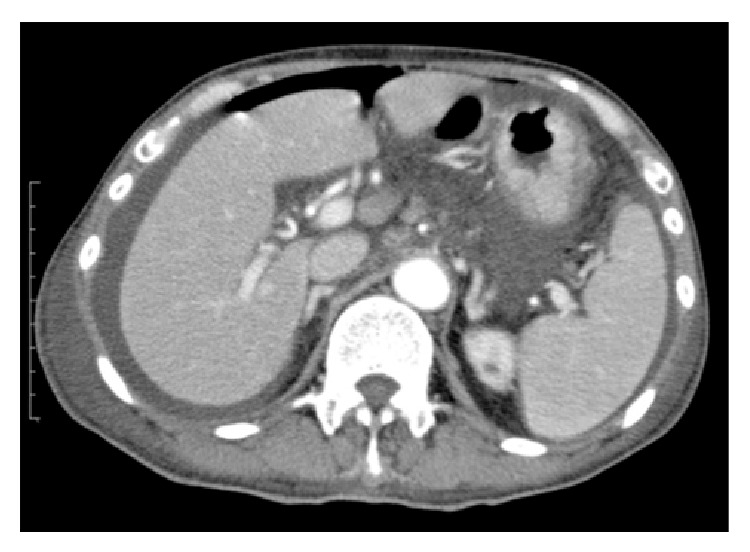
Detection of free air in the patient with thyroid storm, a 55-year-old Japanese male. Contrast-enhanced abdominal CT shows the presence of free air in the surface of the liver associated with ascites.
